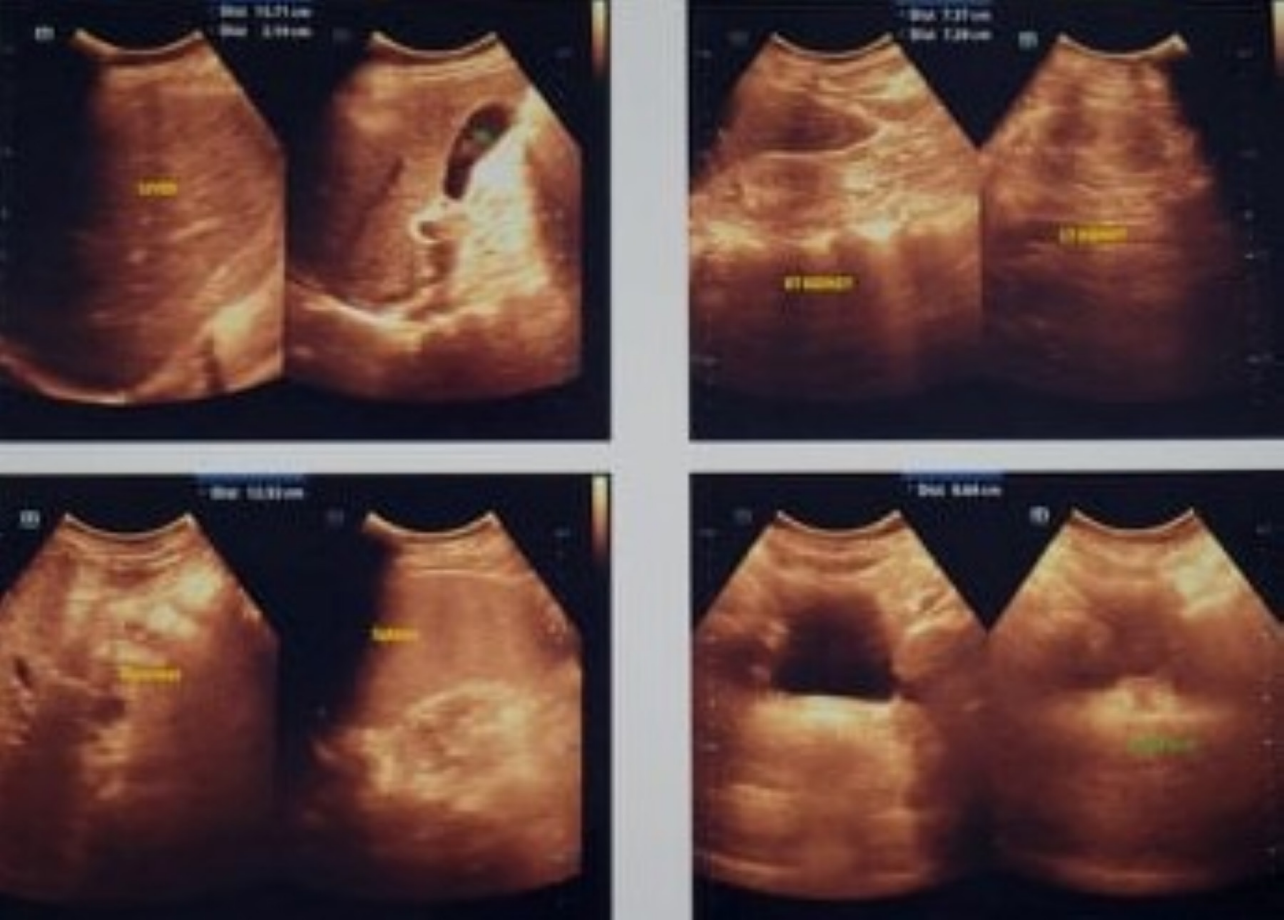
Ultrasonography of the abdomen showing bilateral renal parenchymal disease, fatty change in the liver (grade 2), and cholelithiasis.
